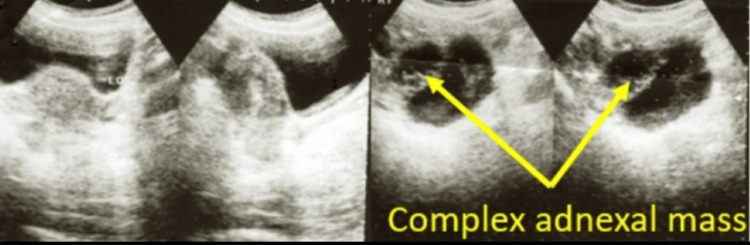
Tubal pregnancy seen on transvaginal sonography
